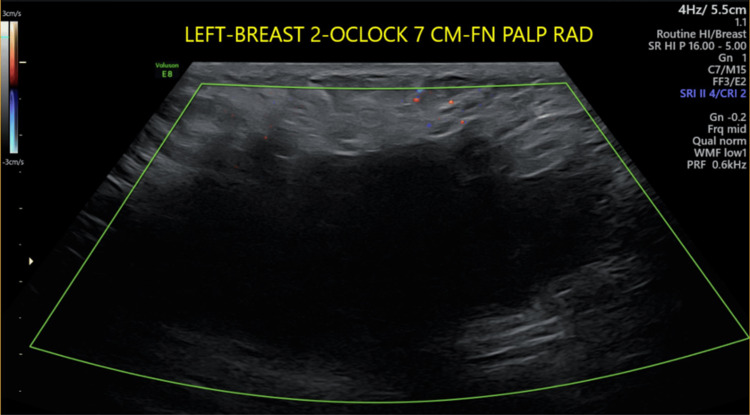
Color Doppler ultrasound of the breast Color Doppler ultrasound image showing no vascular flow to the left breast irregular hypoechoic mass with angular margins and posterior acoustic shadowing.
